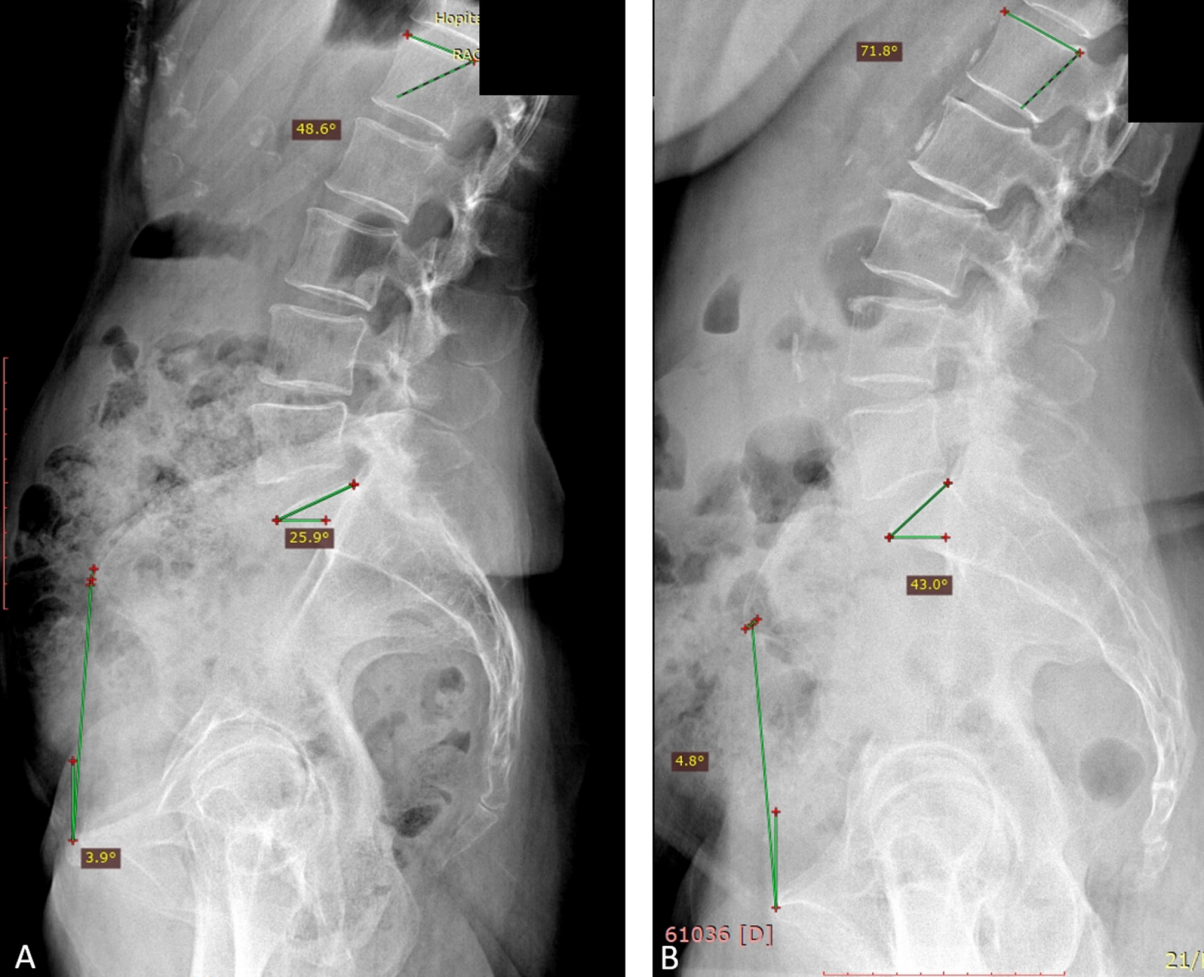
Type of lumbar shape in patients with low pelvic incidence (PI) in lateral standing radiographs. a) Patient with low PI and low lordosis. PI = 38°, spinopelvic tilt (SPT) = -4°, lumbar lordosis (LL) 49°, sacral slope (SS) 26°, and lumbar apex at level L5. b) Patient with low anteverted PI 39°, SPT 5°, LL 71°, SS 42°, and lumbar apex at level L4.
